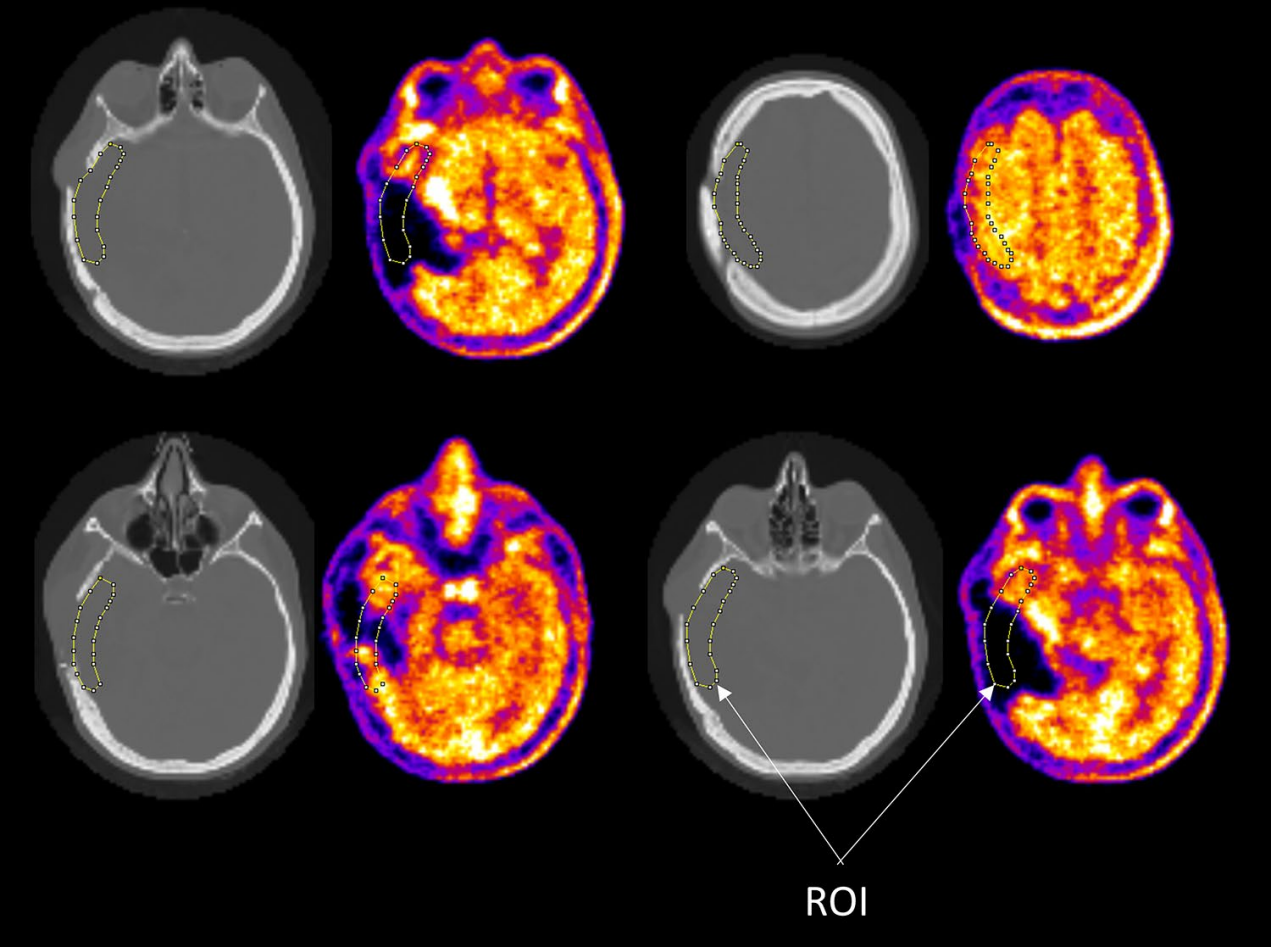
Four representative images (CT (left) and PET (right)) of a METH patient with superimposed ROIs for PET quantification in the anatomically altered region
